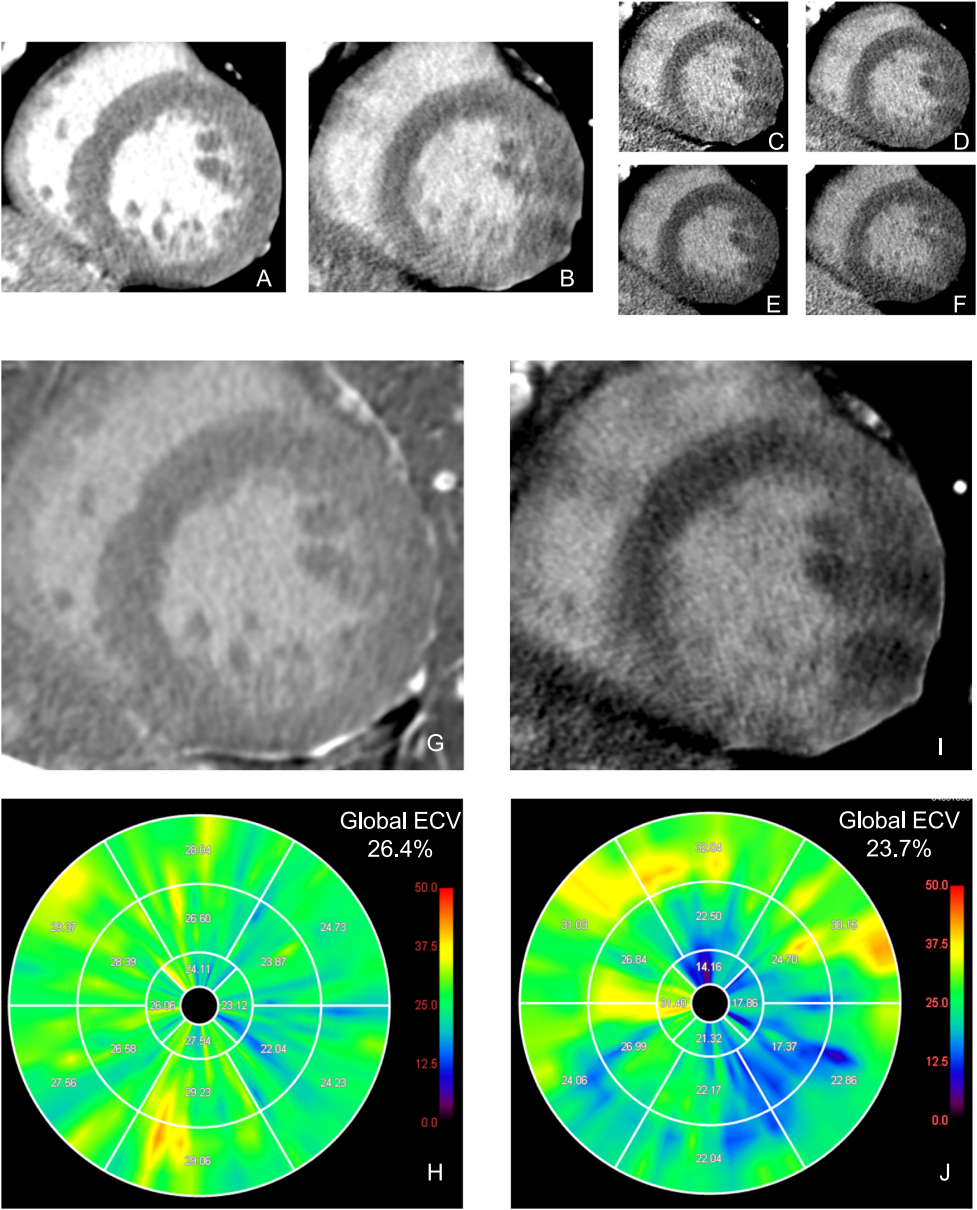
CT-LE in a 75-year-old man without history of myocardial infarction undergoing percutaneous coronary intervention for angina pectoris. CT-LE images include shuttle mode (A) and DE mode images: mono-plus 40 keV (B), 40 keV (C), 60 keV (D), 70 keV (E), 80 keV (F). A short-axis subtraction image (G) and a polar map of ECV (H) derived from subtraction images and an iodine-specific image (I) and a polar map of ECV (J) derived from iodine-specific images are also presented. Streak artifacts are more pronounced in DE mode than in shuttle mode, resulting in a larger variation in segmental ECV. CT-LE, CT late enhancement; DE, dual-energy; ECV, extracellular volume
